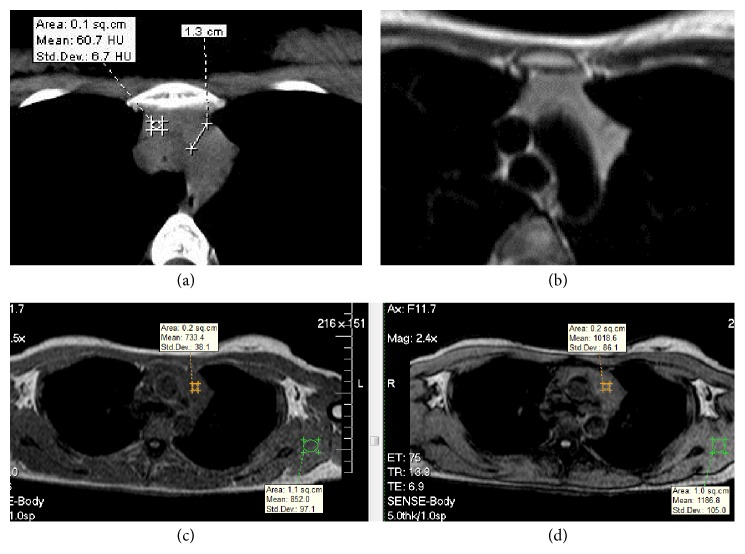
(a) CT image, (b) T2-weighted MRI, and (c) in-phase and (d) opposed-phase images presenting the gland without adipose tissue with CSR 1.0.
